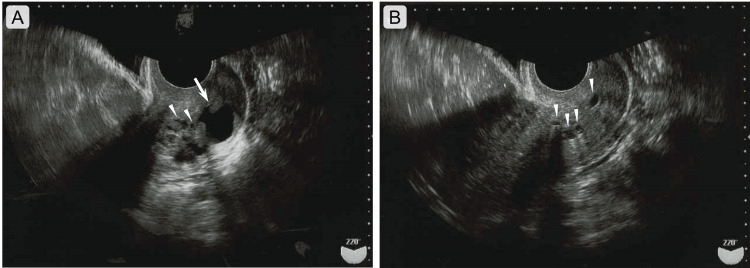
Transvaginal ultrasonographic images of multiple Nabothian cysts and tunnel clusters in the uterine cervix. Large, small, low-echoic lesions, suggestive of various-sized cysts, are present in the uterine cervix. The large cyst is indicated by an arrow in (A). The small cystic lesions are shown using arrowheads in (A)-(B). The scale bars indicate photographs.
